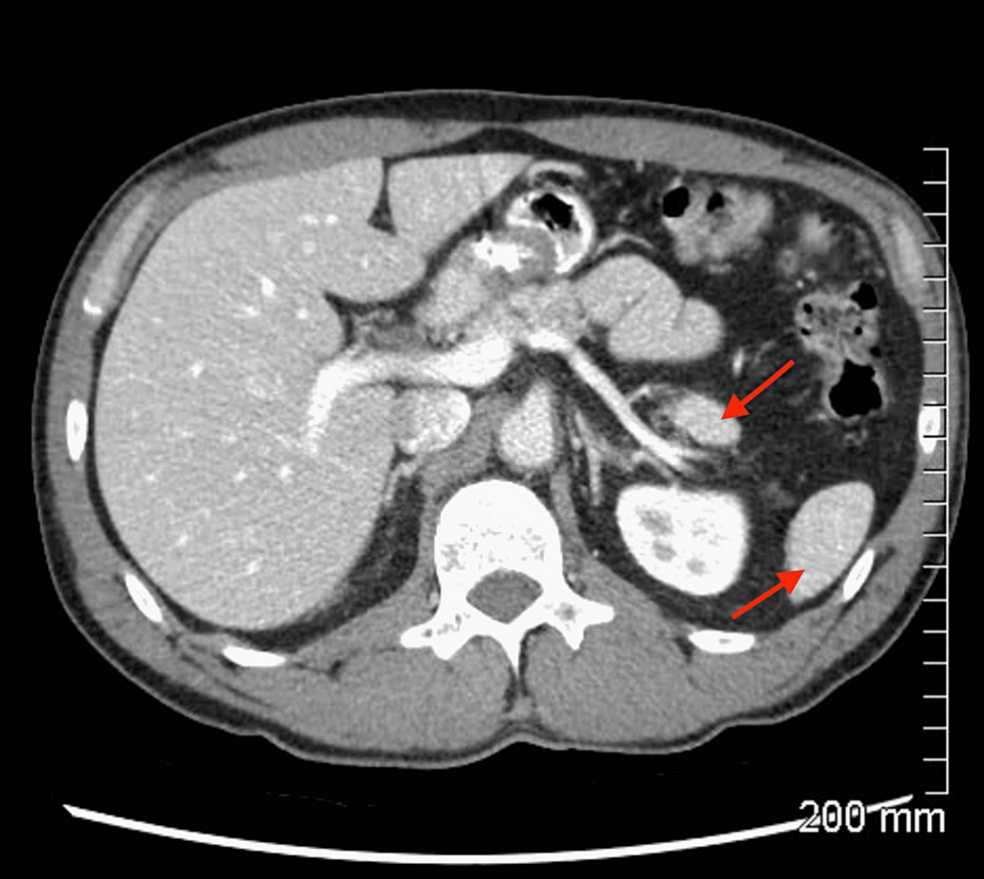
Abdominal CT with contrast showing a hyperintense, enhancing 1.9 cm soft tissue mass within the pancreatic tail distinct from the surrounding parenchyma. Arrows indicate the pancreatic mass and spleen.
